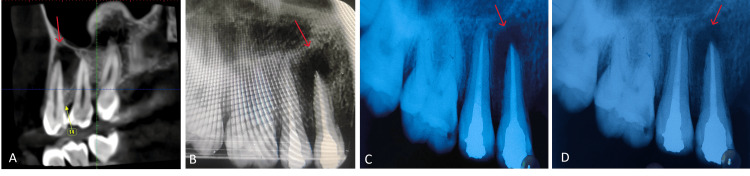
(A) Cone beam computed tomography of tooth number 14, 15; (B) post-obturation and final restoration with tooth number 14, 15; (C) six-month follow-up radiograph; and (D) 12-month follow-up radiograph.
